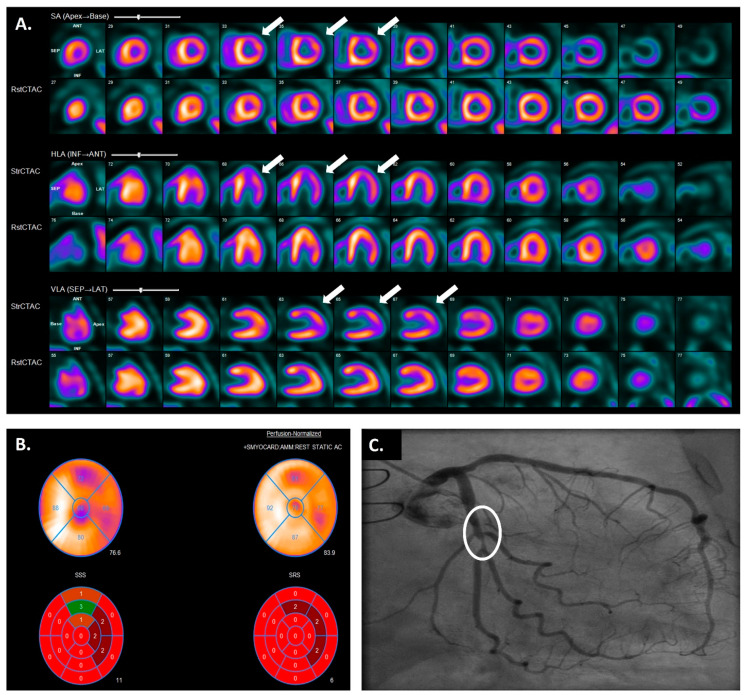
Moderate to large reversible perfusion defect (white arrows) in the anterior and inferolateral wall showed using positron emission tomography (A) and computed tomography cardiac perfusion test (B). Coronary allograft vasculopathy was confirmed with invasive coronary angiography; this study showed a severe obstruction (white circle) in the mid circumflex artery (C).
